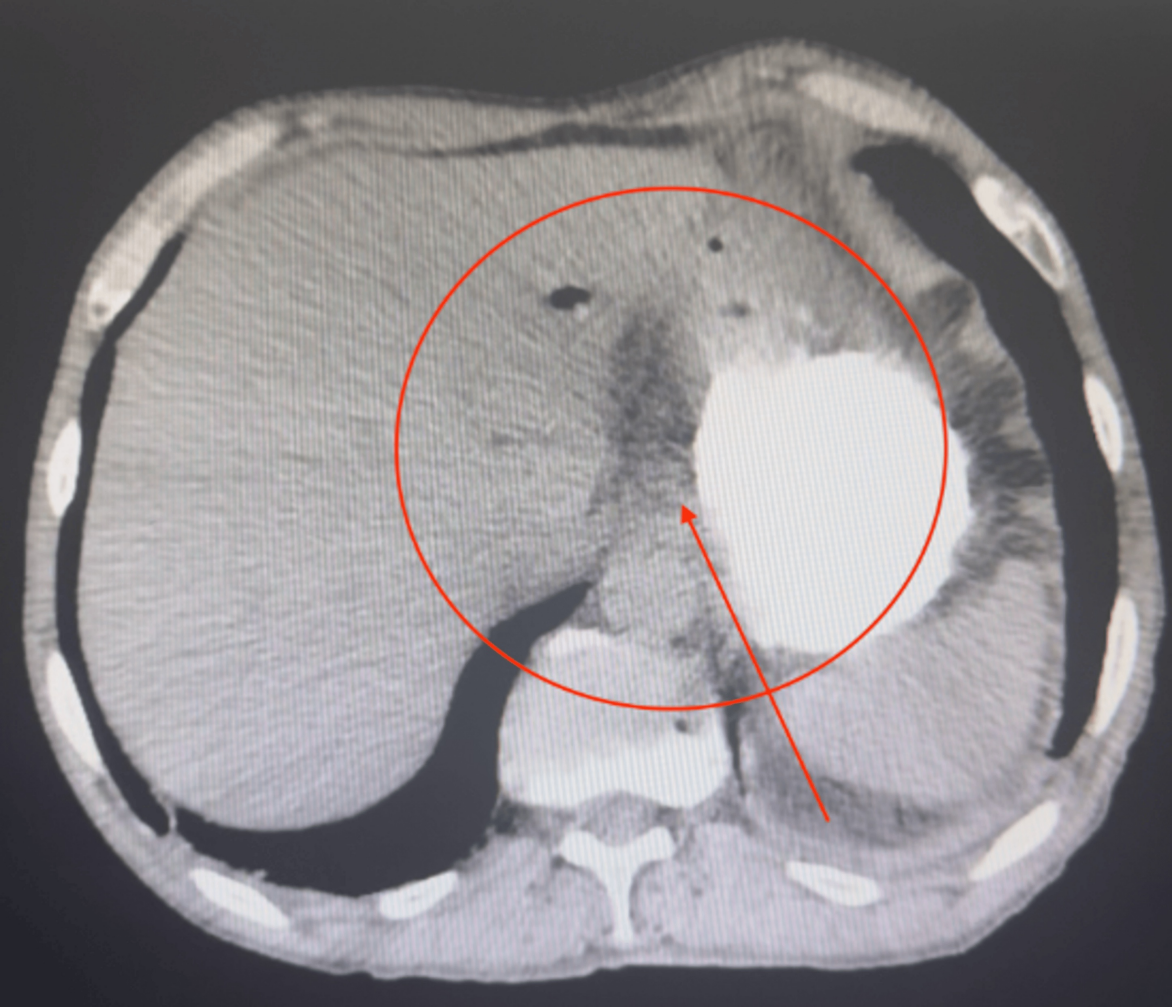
Axial CT scan of the abdomen with oral contrast showing free intraperitoneal air in the subhepatic space (highlighted by the red circle and arrow), adjacent to the anterior wall of the gastric body. Although the ingested wooden toothpick is not visible due to its radiolucency, this finding raised suspicion of a contained gastrointestinal perforation, leading to exploratory laparotomy.
